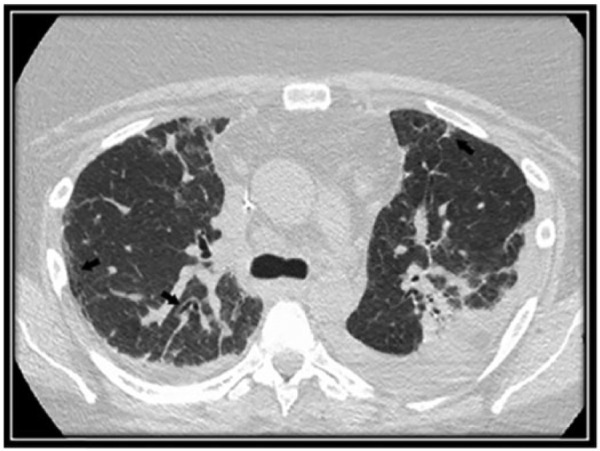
Chest computed tomography scan after therapy. Resolution of nodular infiltrates with residual traction bronchiectasis and fibrosis from known nonspecific interstitial pneumonitis.
